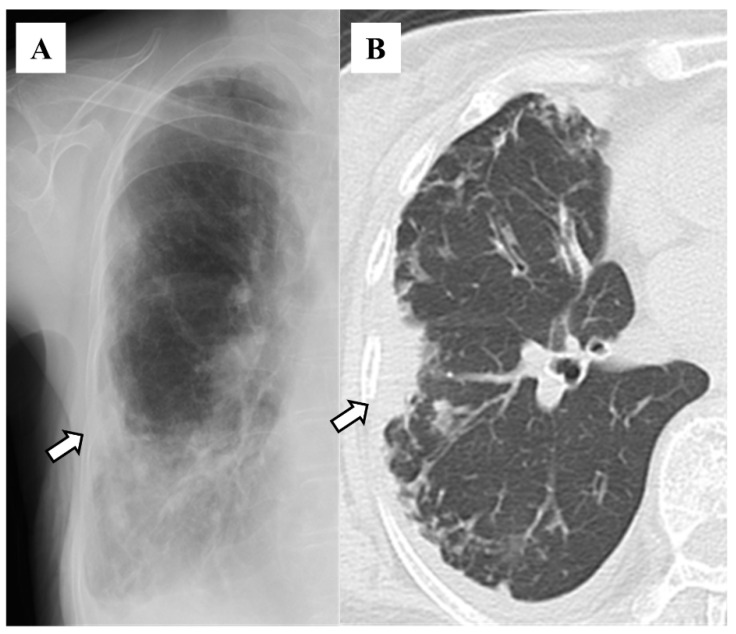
Pleural thickening on chest X-ray (A) and high-resolution computed tomography (B). White arrows indicate the same lesions within the patient.
